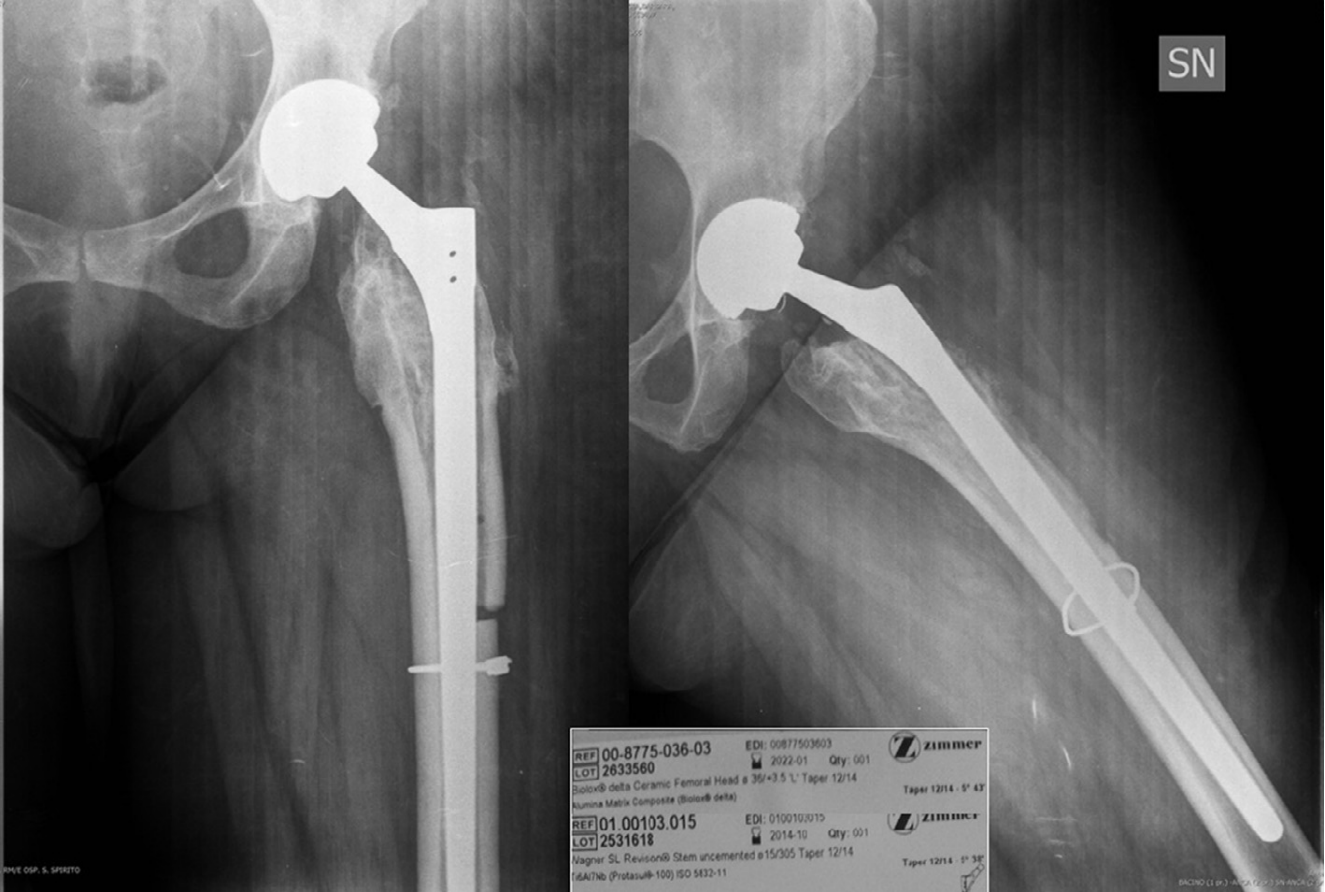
Postoperative X-ray after 2nd revision. The stem choice was a monolithic Wagner revision implant with the following details: femoral head 36 + 3.5, Lima Wagner Sl revision stem. The Wagner stem is already hole.
